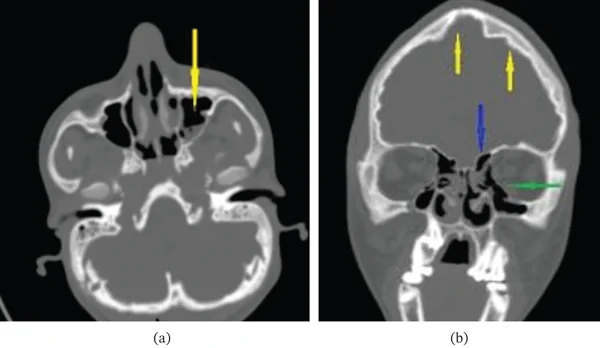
CT paranasal sinuses showing maxillary (a, yellow arrows), sphenoid, frontal and ethmoid sinusitis (b, blue and green arrows). Variable thickness of inner and outer tables with diffuse indentation giving a beaten‐copper appearance to the skull (b, yellow arrows).
